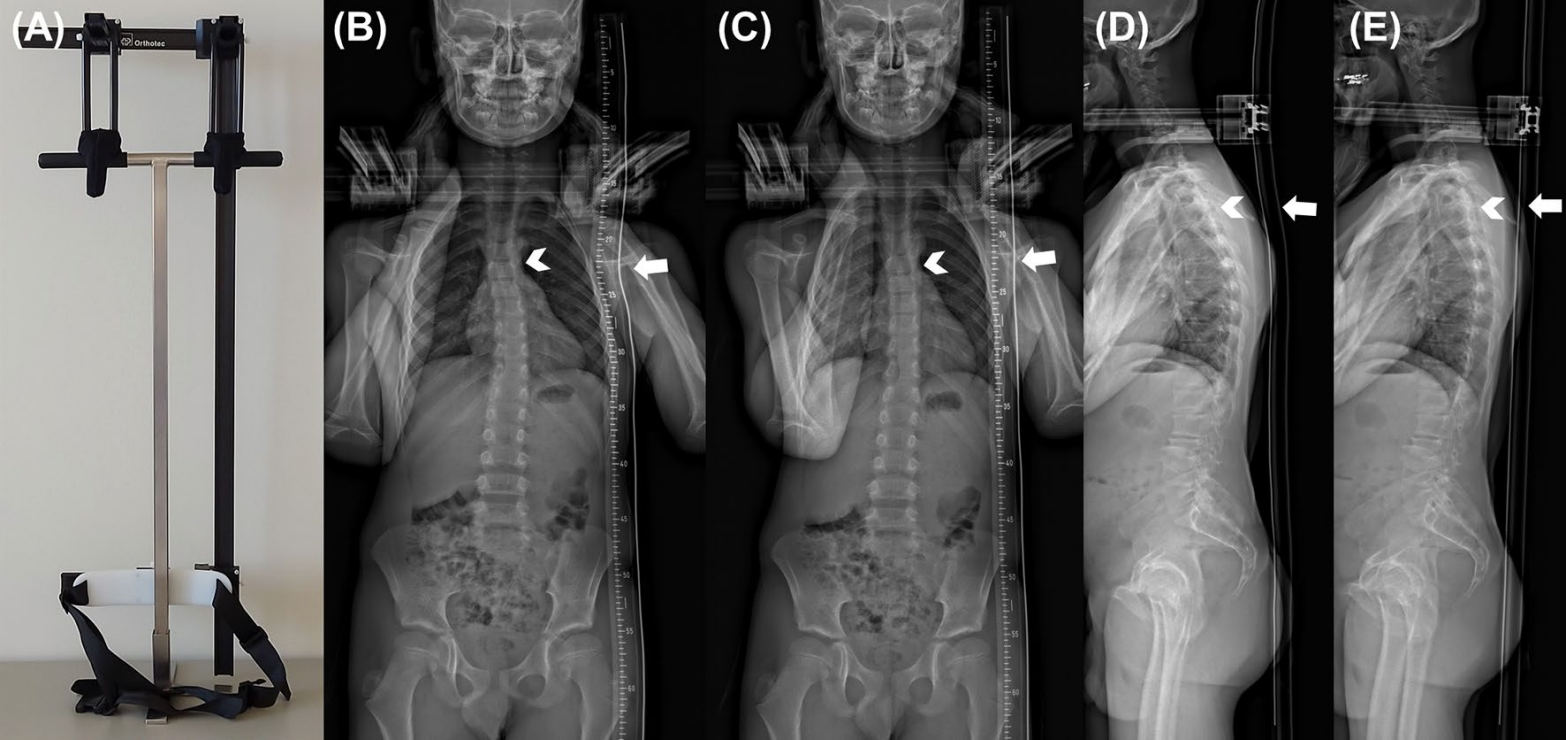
Hardware setup and X-ray images. (A) Picture of the developed setup with a metal wire as reference device. (B) image of a male 8-years old patient, where the metal wire is not straight (arrow), but bent at the height of the upper thoracic spine. These motion artifacts resemble a slight scoliosis at the level of Th5 / Th6 (arrow head). (C) image of the same patient after repeating the acquisition. The metal wire is straight (arrow) and no scoliotic change is found (arrow head). (D) image of a 40-years old male patient, where the metal wire is mildly bent (arrow) at the level of the upper thoracic spine, resembling a pronounced kyphosis (arrow head). (E) image of the same patient after repeating the acquisition. The metal wire is straight (arrow), and the upper thoracic spine is less kyphotic (arrow head).
